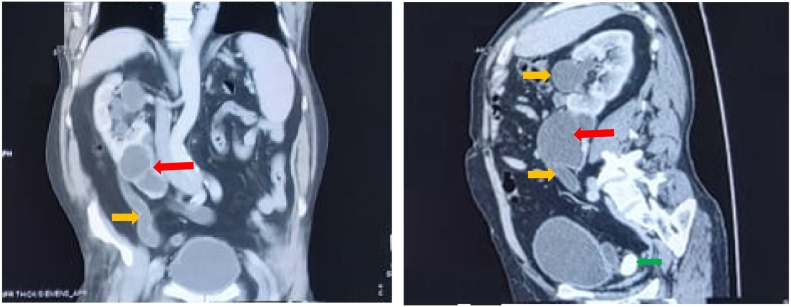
Frontal and sagittal views of CT showing crossed ectopic fused left kidney. The ectopic left kidney (red arrow ), with laminated parenchyma, is fused to the inferior pole of the right kidney, and was responsible of the obstruction and distension of the right ureter and pelvicalyceal (yellow arrow ). Image of ureteral calculi is shown by green arrow .
